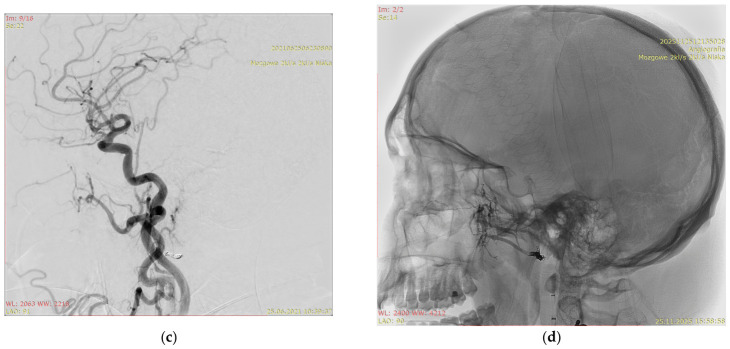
Left-sided tentorial dural arteriovenous fistula (dAVF) in Patient X. (a) Pre-treatment image of the dAVF, (b) microarteriography of the lesion, (c) post-treatment vascular image, (d) X-ray image showing the embolic material. The term “mózgowe 2 kL/s, 2 kL/s niska” translates as “Cerebral 2 fps, 2 fps Low”.
